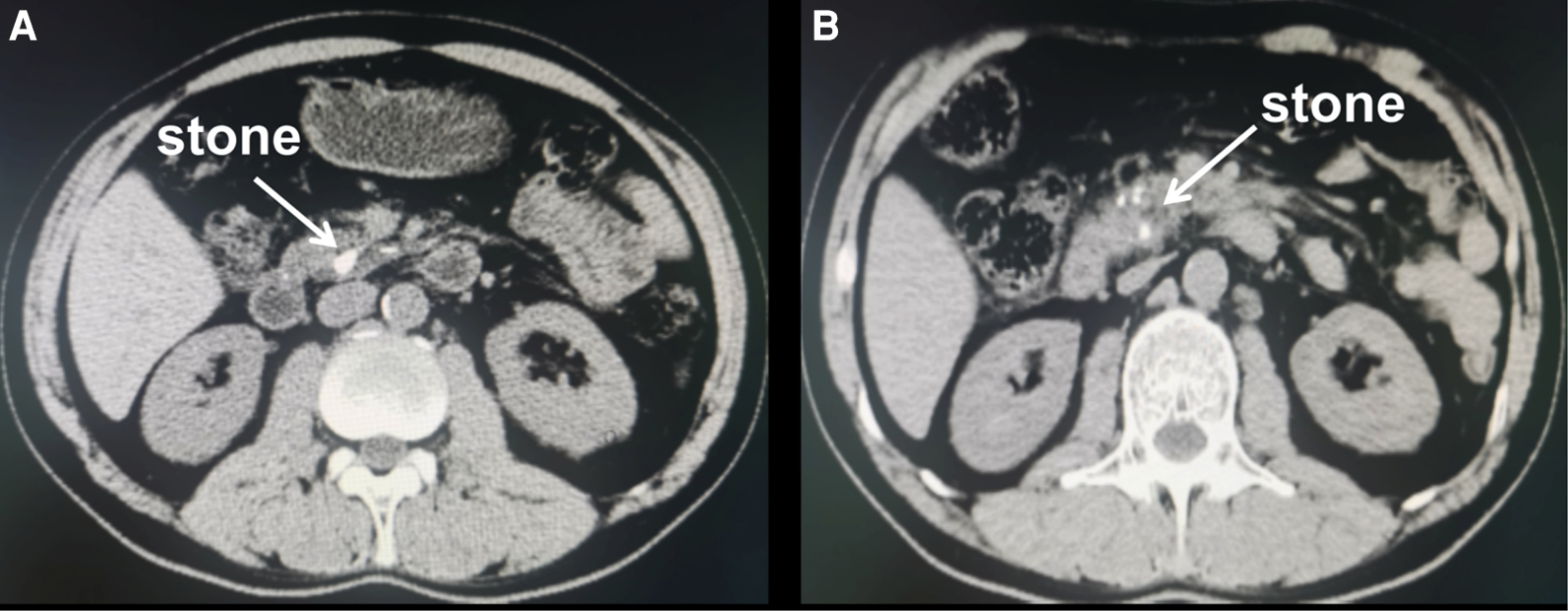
Computed tomography scan of the patient's abdomen. (A,B) CT showing acute pancreatitis and large calculi in the pancreatic duct (white arrow).
